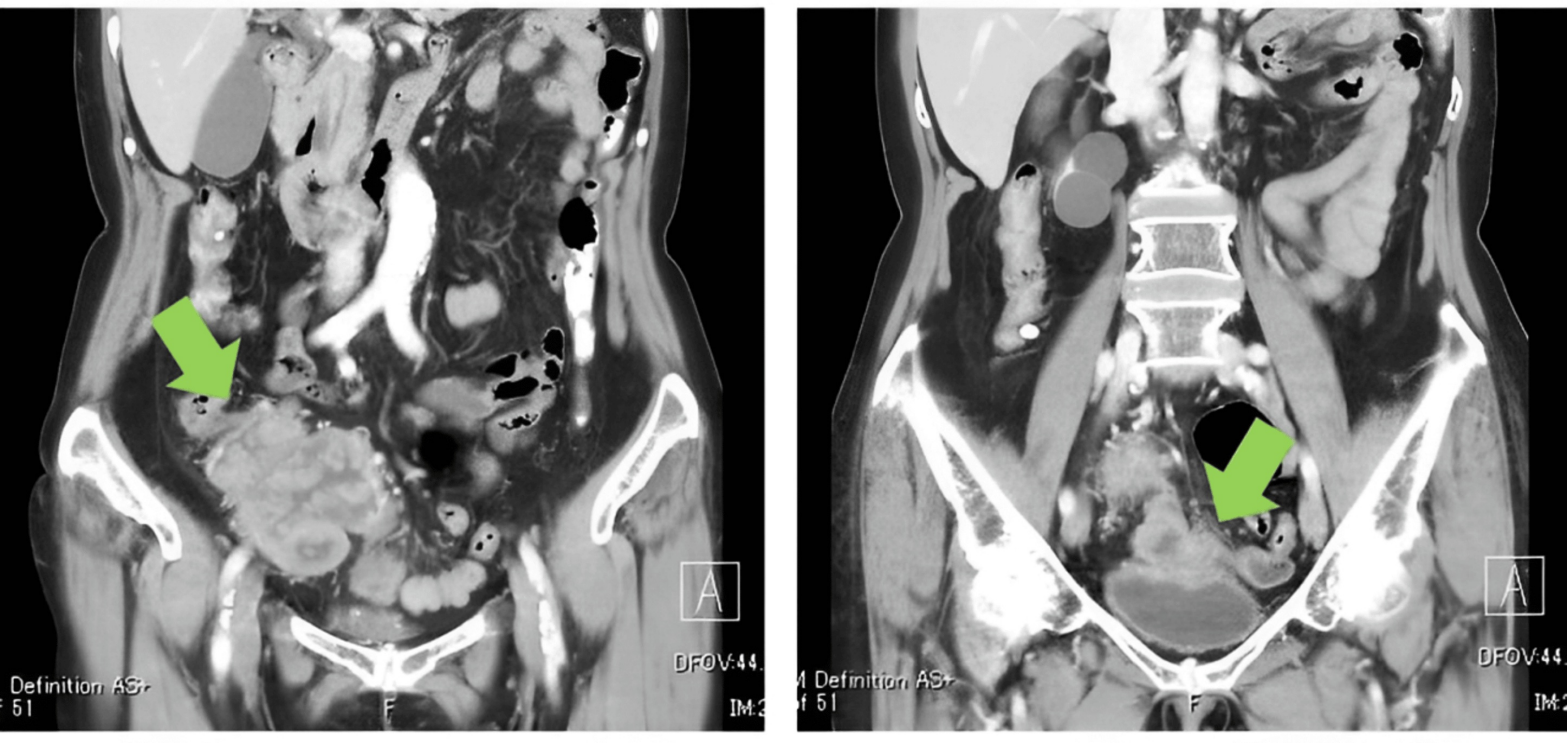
A contrast-enhanced CT scan A contrast-enhanced CT scan demonstrated a fistulous connection between the bladder and the cecum, indicative of an enterovesical fistula (EVF) caused by a malignant tumor (green arrow).
